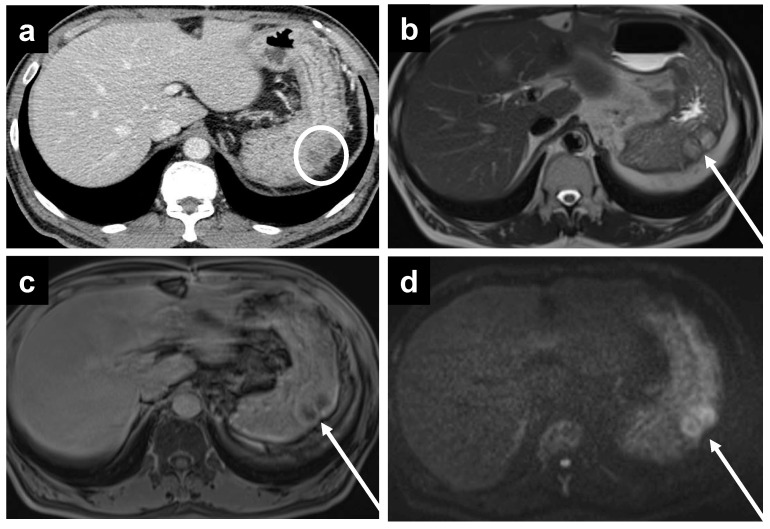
A 67-year-old male with metastatic colonic adenocarcinoma and gastric serosa peritoneal deposits. The deposits are not as well seen on (a) CT, as denoted by the white circle, but are more apparent on MRI, including the (b) T2W, (c) delayed, and (d) DWI sequences secondary to the superior soft tissue contrast.
